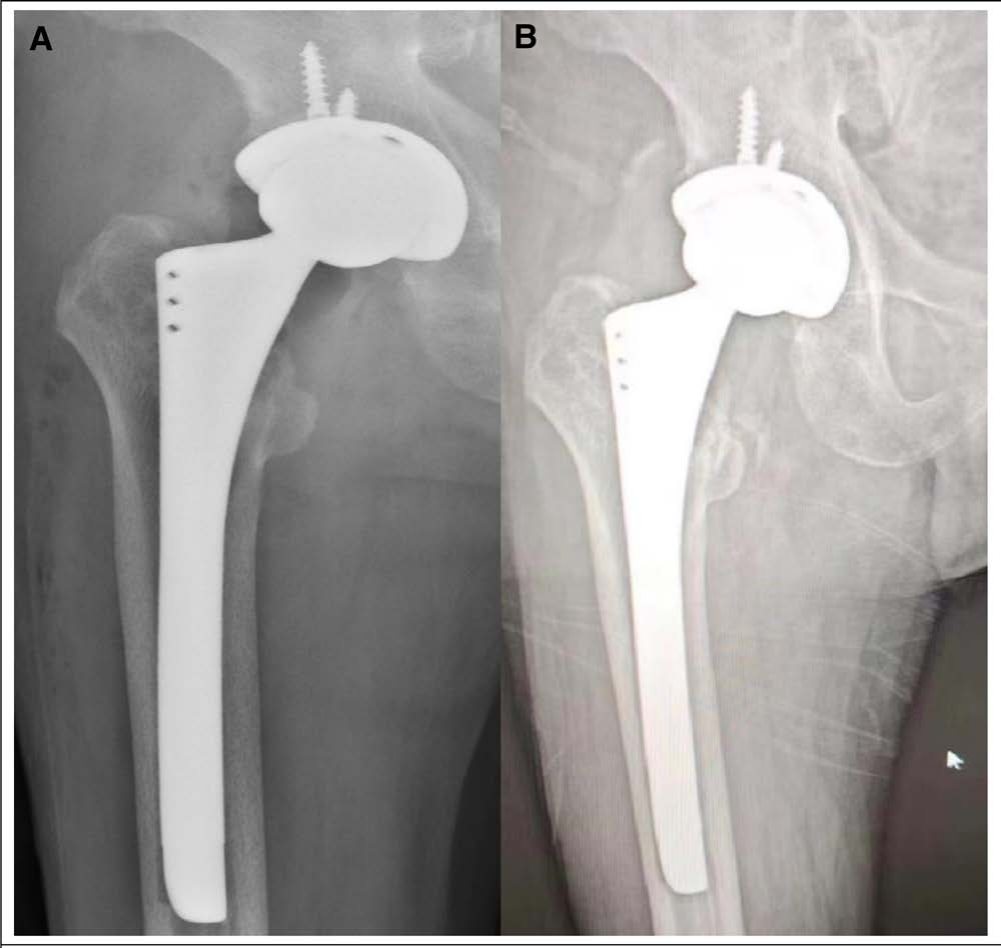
(A) The postoperative anteroposterior X-ray showed total hip arthroplasty for right femoral neck fracture. (B) Follow-up X-ray of hip joint demonstrated stable conditions of bone and hip prosthesis.
